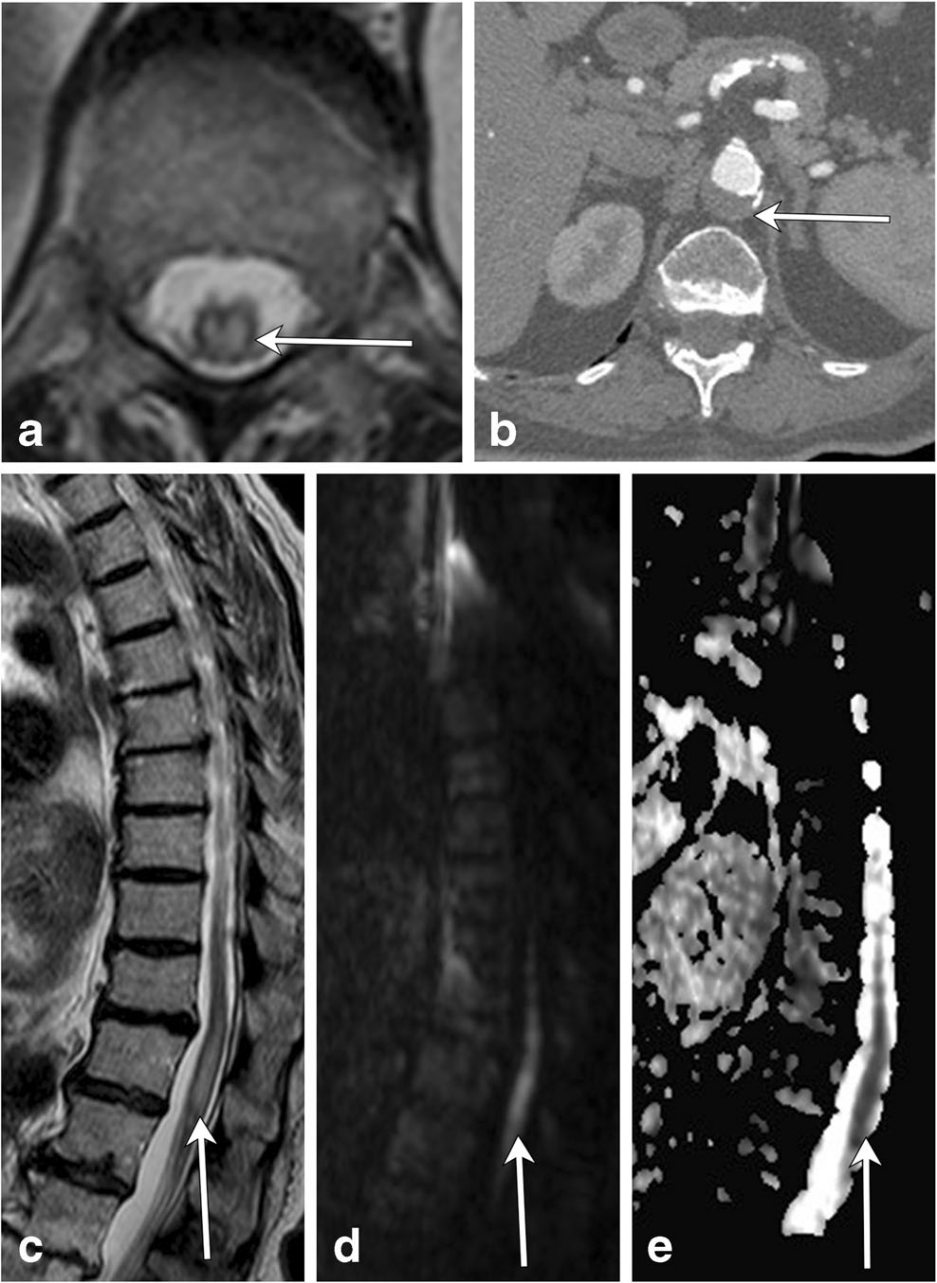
Spinal cord infarction. An 82-year-old female with hypertension and hyperlipidaemia presents with acute bilateral lower extremity weakness. a Axial T2 image demonstrates a large cross-sectional area of hyperintensity (arrow) within the thoracic cord. b Axial CT angiogram image shows mural thrombus within the aorta (arrow) at the same level of the spinal cord findings with no appreciable filling of the lumbar arteries. Sagittal T2 (c), DWI (d) and ADC (e) images reveal a long-segment intramedullary lesion in the lower cord (arrows) that is T2 hyperintense and has restricted diffusion
